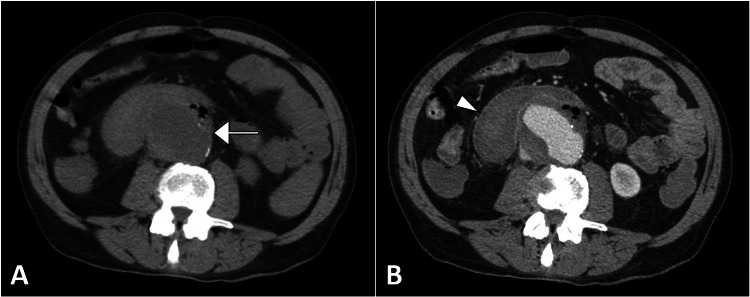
The axial CT image in the non-contrast phase (A) shows an abdominal aortic aneurysm (arrow). The contrast-enhanced image (B) highlights a faint contrast enhancement of the small bowel loop (arrowhead) adjacent to the aneurysm, confirming the presence of an aorto-enteric fistula. CT: computed tomography.
